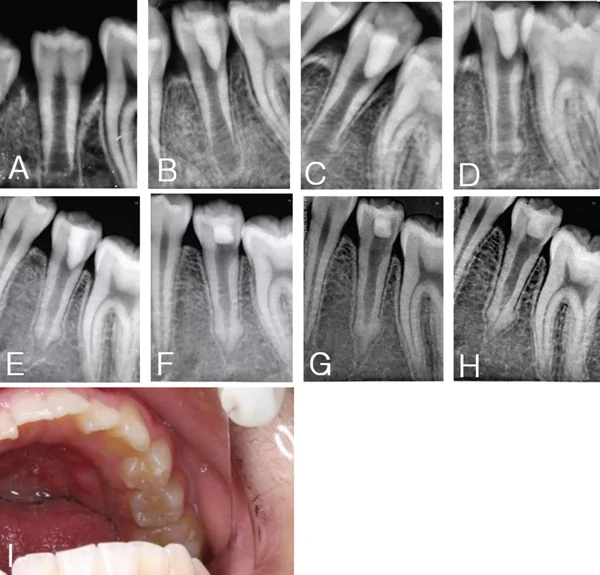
(A) Preoperative periapical radiograph; (B) 1‐month postoperative radiograph; (C) 3‐month postoperative radiograph; (D) 6‐month postoperative radiograph; (E) 2‐year postoperative radiograph; (F) 2.5‐year postoperative radiograph; (G) 3‐year postoperative radiograph; (H) 4‐year postoperative radiograph; and (I) intraoral photographs at the 4‐year follow‐up.
